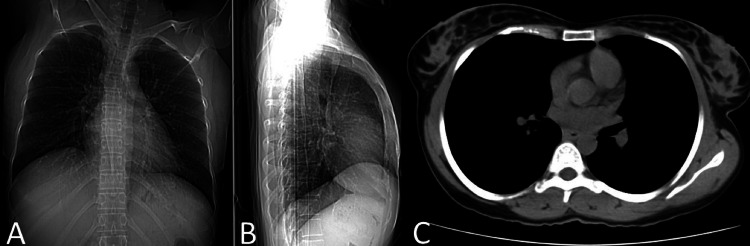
Chest radiograph and HRCT scan image showing no evidence of lung metastasis (A) Posteroanterior view of the chest radiograph. (B) Lateral view of the chest radiograph. (C) Axial section of HRCT. HRCT - High-resolution computed tomography
